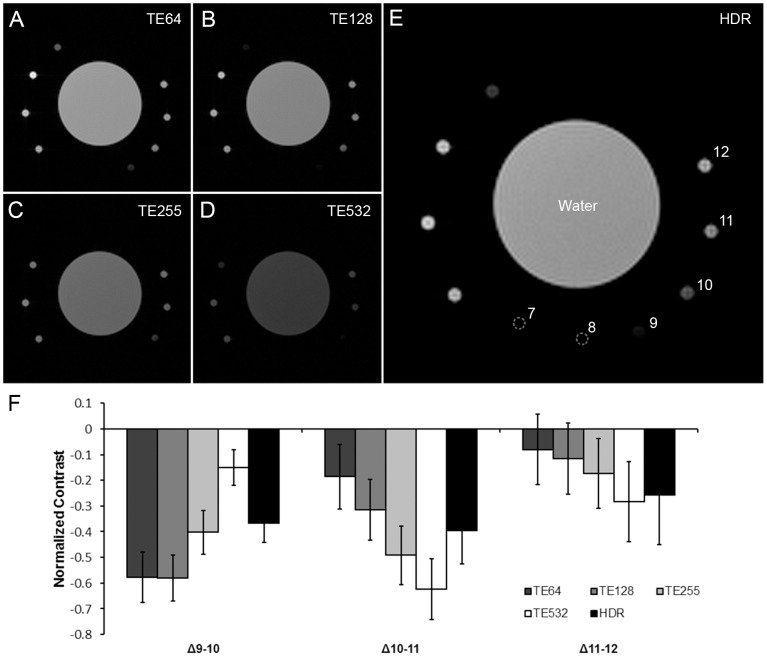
T_2_-weighted HDR-MRI. A series of images were acquired with constant T_R_ at 4000 ms and T_E_ at (A) 63.81 ms (B) 127.62 ms (C) 255.24 ms and (D) 531.75 ms. Images A–D were computationally merged by the HDR algorithm to generate the (E) HDR image. HDR-MRI accurately captured the intensity difference between samples 9, 10, 11, and 12, which was not achieved in any of the source images. This was shown quantitatively by contrasts normalized against water (F). Image A was poor at differentiating samples 10–12, B and C were poor at differentiating samples 11 and 12, and D was poor at differentiating samples 9 and 10. Error bars represent standard deviation.
